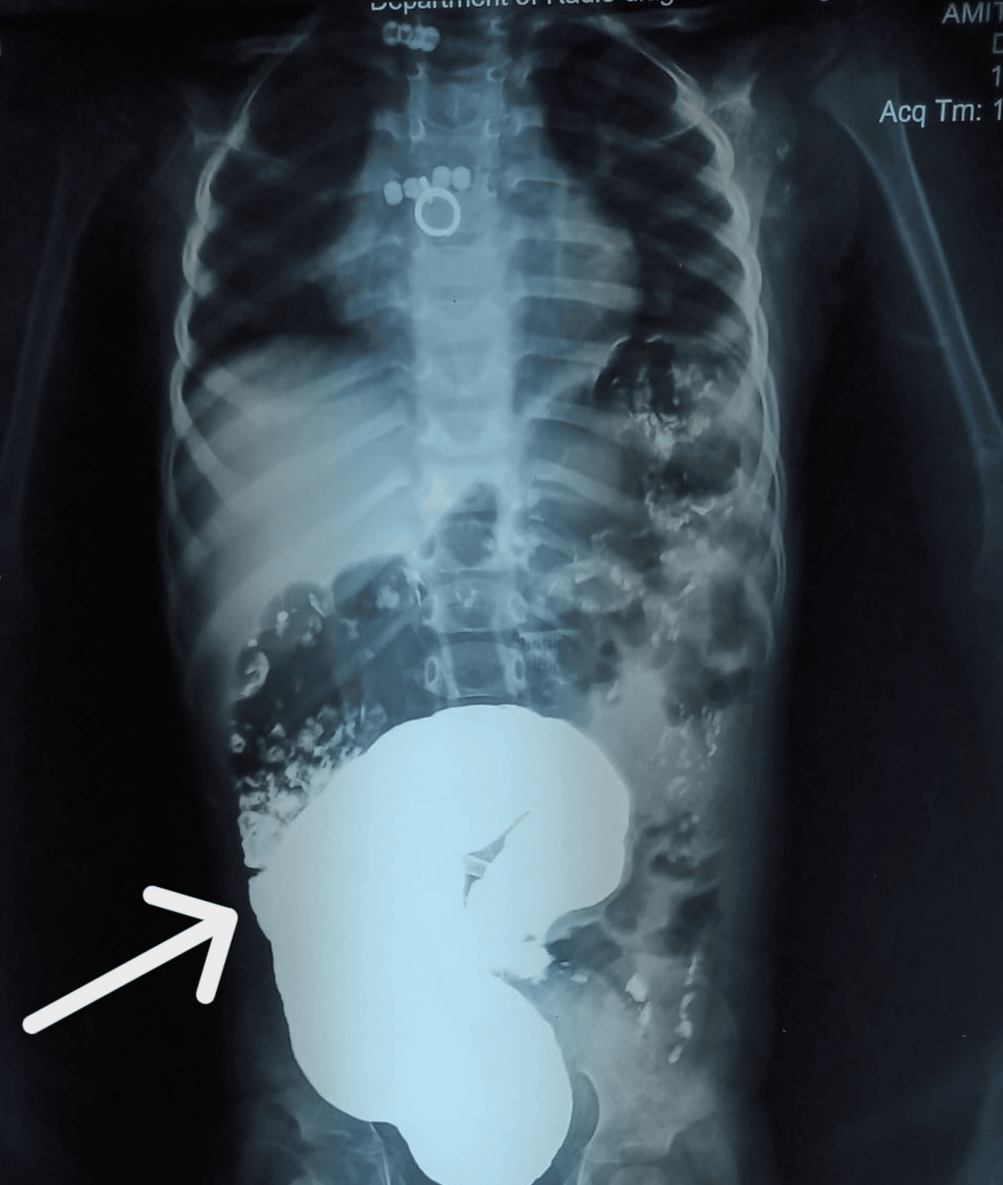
Contrast radiograph demonstrating extrinsic compression of the sigmoid colon Contrast study of the abdomen showing a smooth, well-defined impression over the sigmoid colon (white arrow), produced by an adjacent extrinsic mass effect. The absence of mucosal irregularity or intrinsic narrowing suggests an external compressive etiology rather than primary colonic pathology, consistent with compression by a segmentally dilated ureter.
